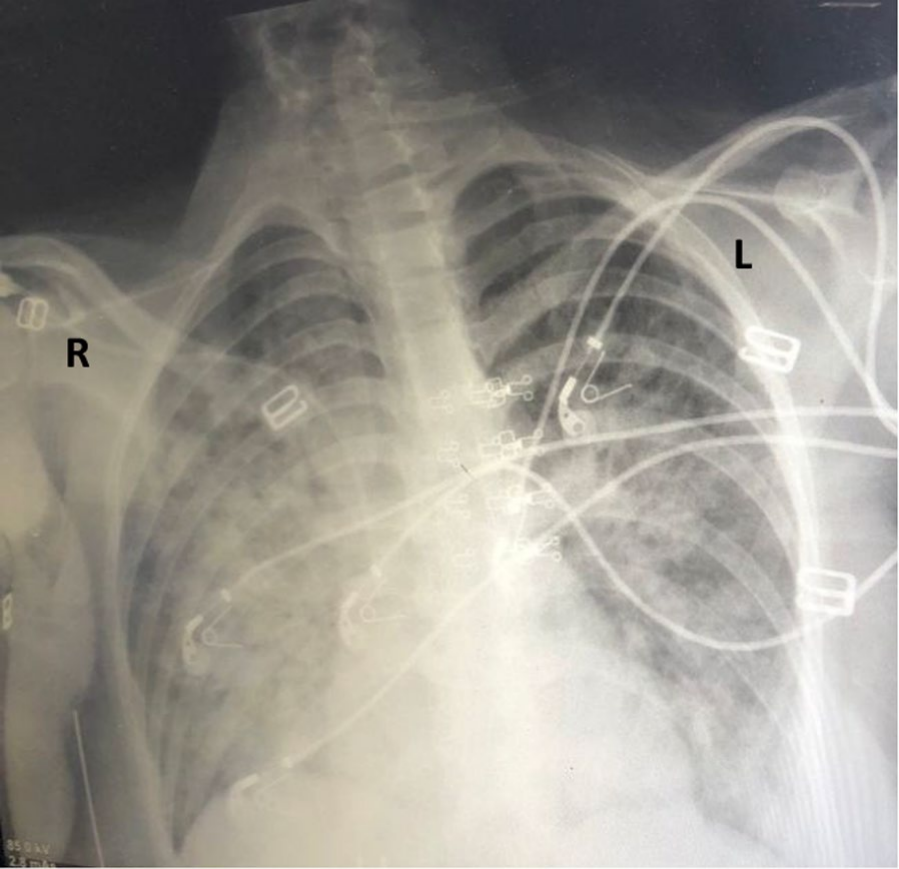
Patient chest X-ray in the ICU showing bilateral heterogenous infiltration and picture of ARDS
